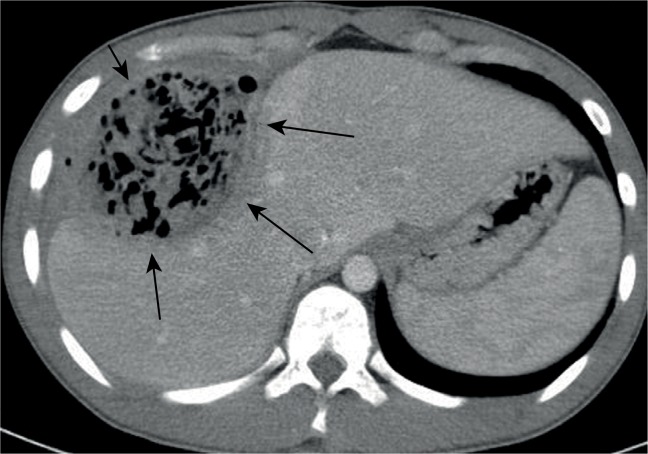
Axial intravenous contrast-enhanced chest CT scan with mediastinal
